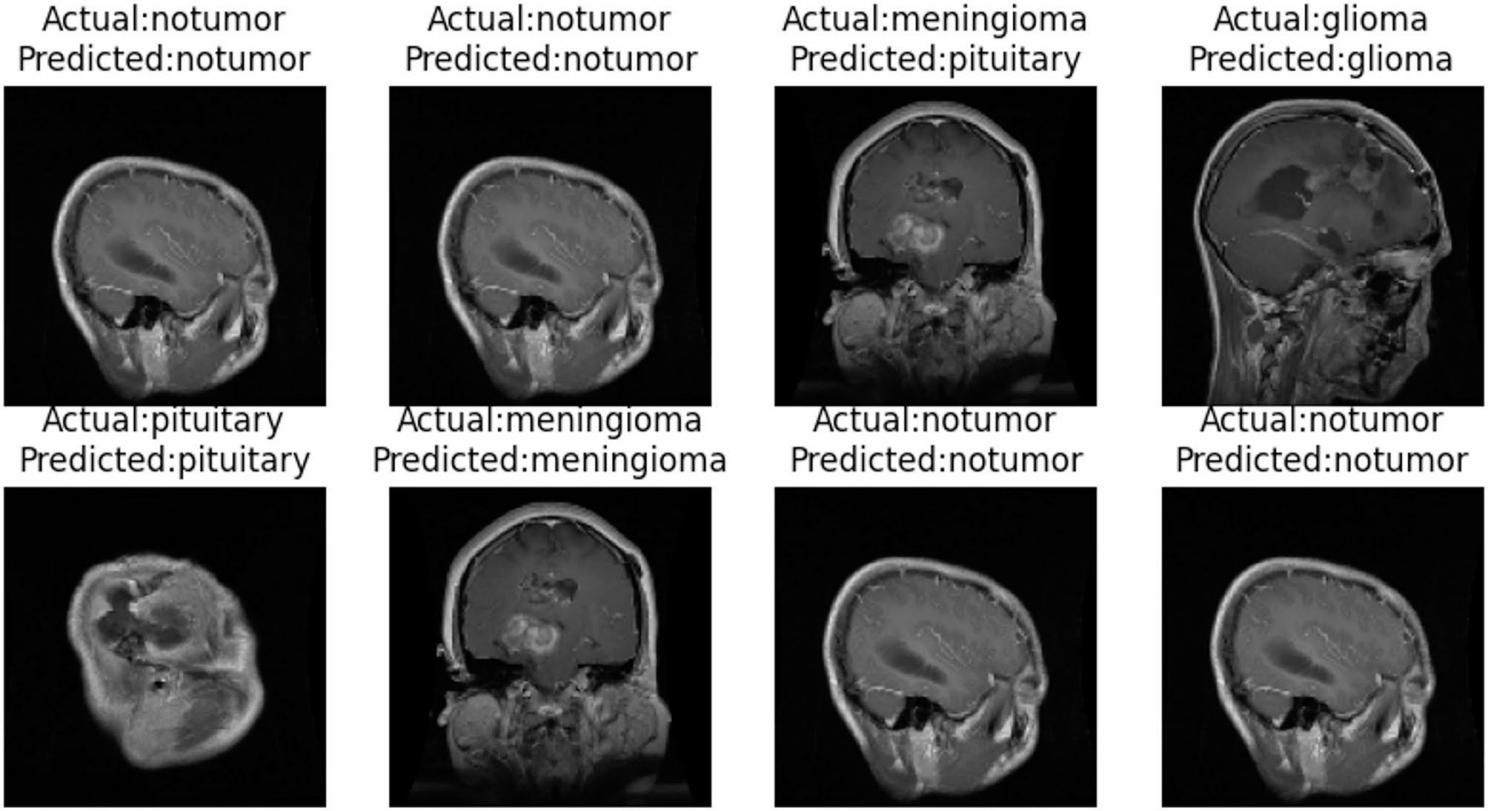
Comparative Study of Actual and Predicted Brain MRI images.
